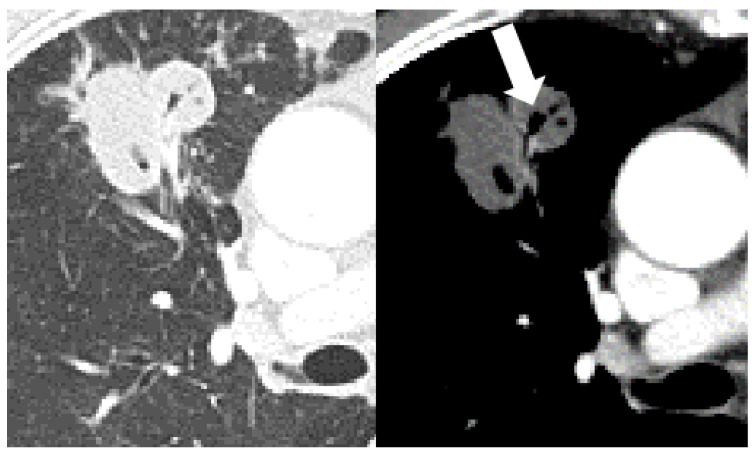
A 70-year-old male with pulmonary tuberculosis and uncontrolled diabetes (HbA1C, 12.3%). The axial CT scan with a lung window setting shows bronchial dilatations within macronodules in the anterior segment of the right upper lobe. Focal bronchial erosion (arrow) is more visible in the mediastinal window setting. Very low-density necrosis of macronodules is also seen.
